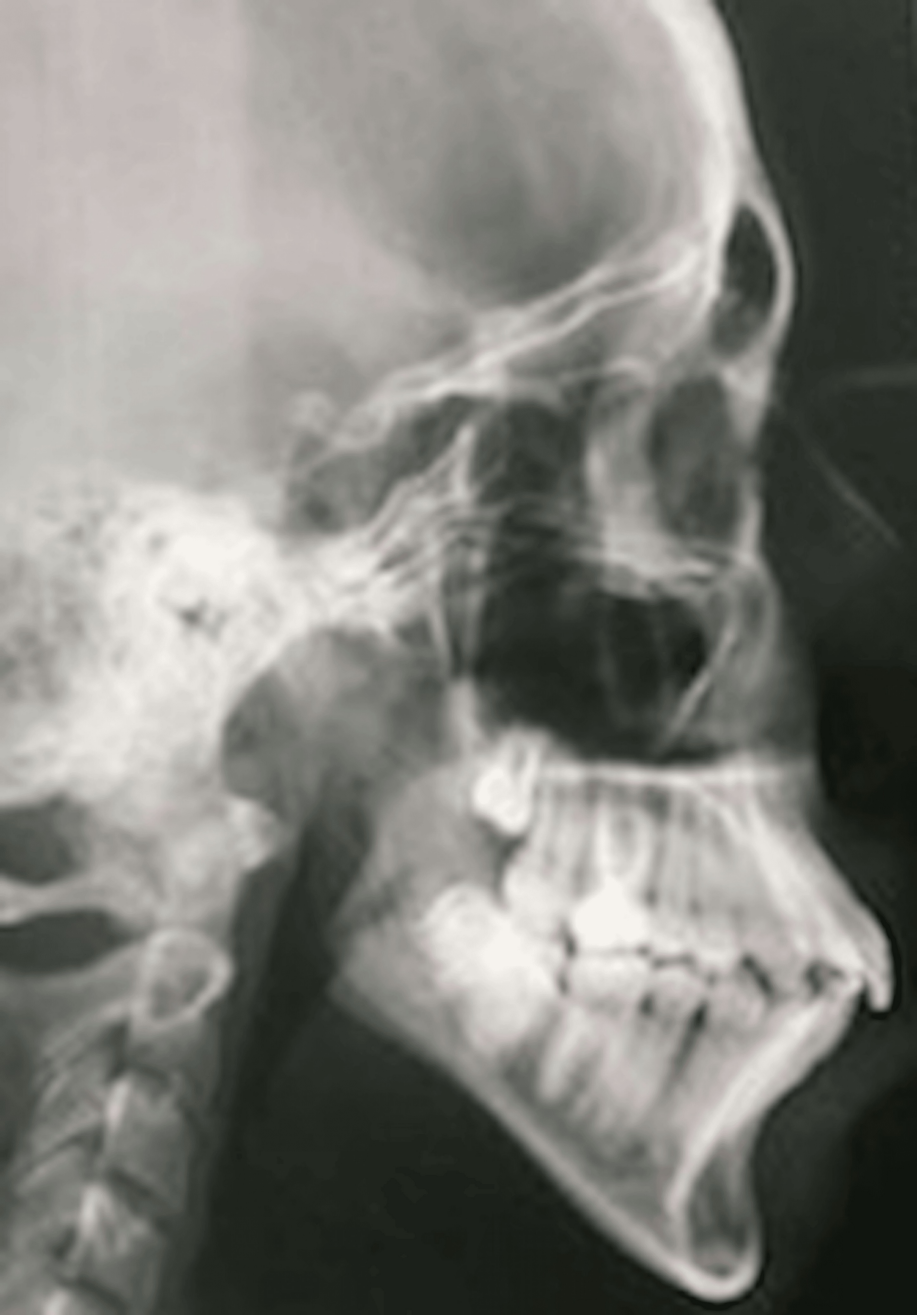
Cephalometry radiograph of female patient in Figure 3 with bimaxillary proclination.
